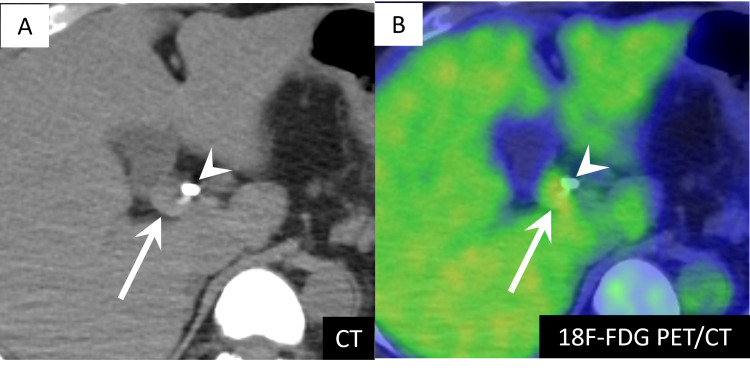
Fluorine-18-fluorodeoxyglucose positron emission tomography (18F-FDG PET)/CT imaging findings. (A) Non-contrast CT shows a nodule (arrow) at the confluence of the three bile ducts. A bile duct stent is inserted in the common bile duct (arrowhead).
